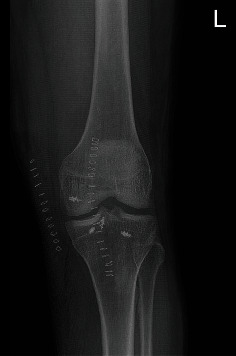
Postoperative A-P radiography of Case 2.
